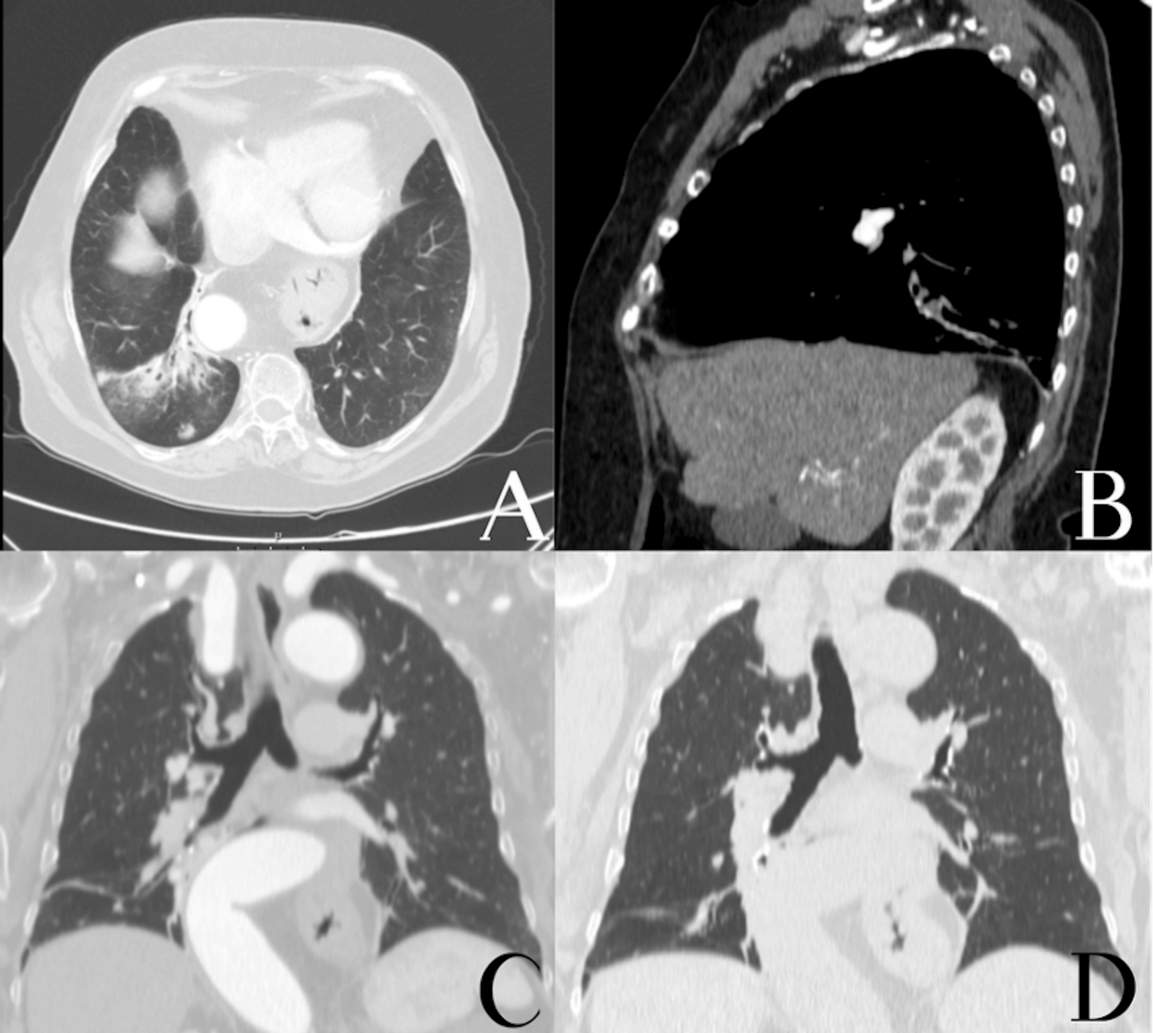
Contrast-enhanced chest computed tomography scan of the patient performed in March 2024. (A)- Axial view showing an area of increased attenuation with traction bronchiolectasis and volume loss in the posterior segment of the right lower lobe, consistent with subsegmental atelectasis and post-obstructive changes. A subpleural nodule with smooth contours and a small calcified focus, measuring approximately 16 mm, is also visible. (B)- Sagittal reconstruction demonstrating the posterior basal right lower lobe atelectatic change. (C-D)- Coronal views highlighting the extent of the atelectatic and fibro-scar alterations in the posterior right lower lobe.
